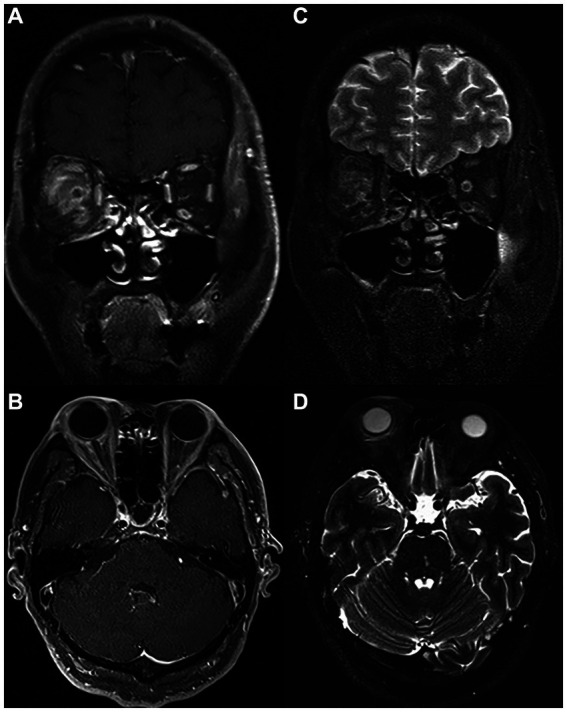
MRI scan of the same patient on the day of PRP injection. Coronal (A) and (B) axial T1 postcontrast images showing successful injection of PRP in the right Tenon capsule near the inner part of the ball with marked postcontrast enhancement. Coronal (C) and (D) axial T2-weighted images showing extensive T2 high signal in the fascia tissue behind the ball of the right eye. PRP, platelet-rich plasma; MRI, magnetic resonance imaging.
